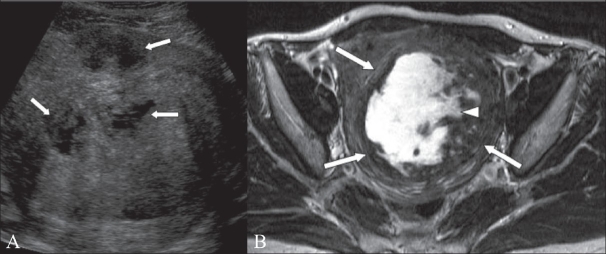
A 46-year-old woman with menorrhagia. TA USG image (A) shows a 7-cm intramural fibroid containing cystic areas (arrows). Axial T2W MRI image (B) shows an 11 × 8 cm fibroid (arrows) containing central high signal, consistent with cystic degeneration (arrowhead)
